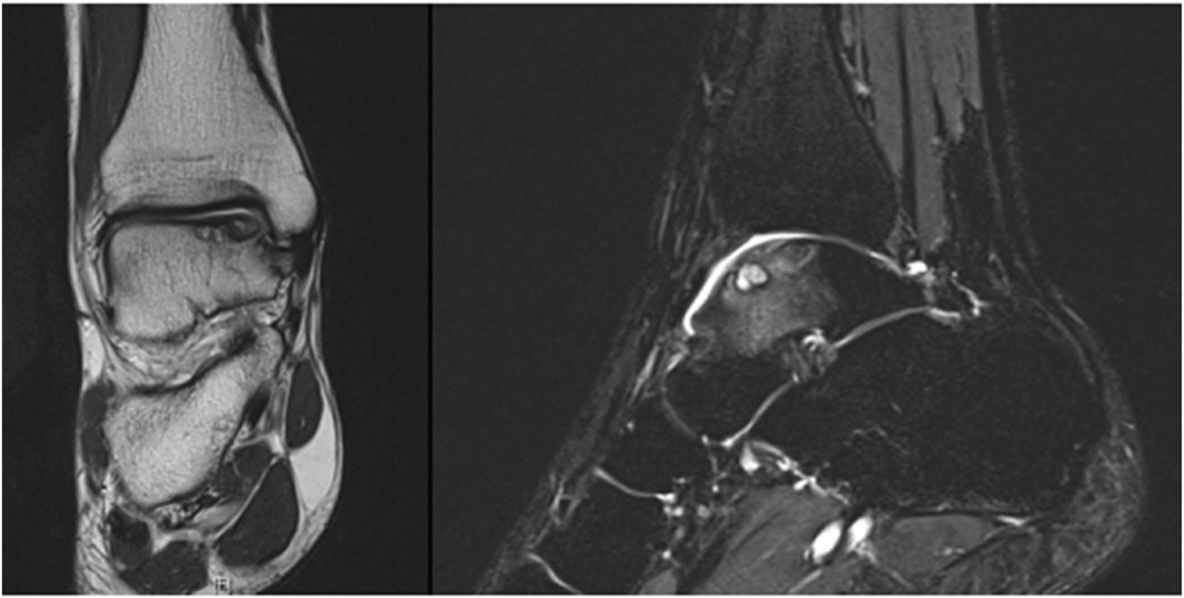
OCL at the medial talar dome is shown on MRI. *Left*: Proton-density-weighted coronal image shows a subchondral cystic area of the medial talar dome with adjacent cartilage defect. *Right*: T2-weighted fat-saturated sagittal image: Subchondral cystic lesion with surrounding bone marrow edema pattern of the talar body
